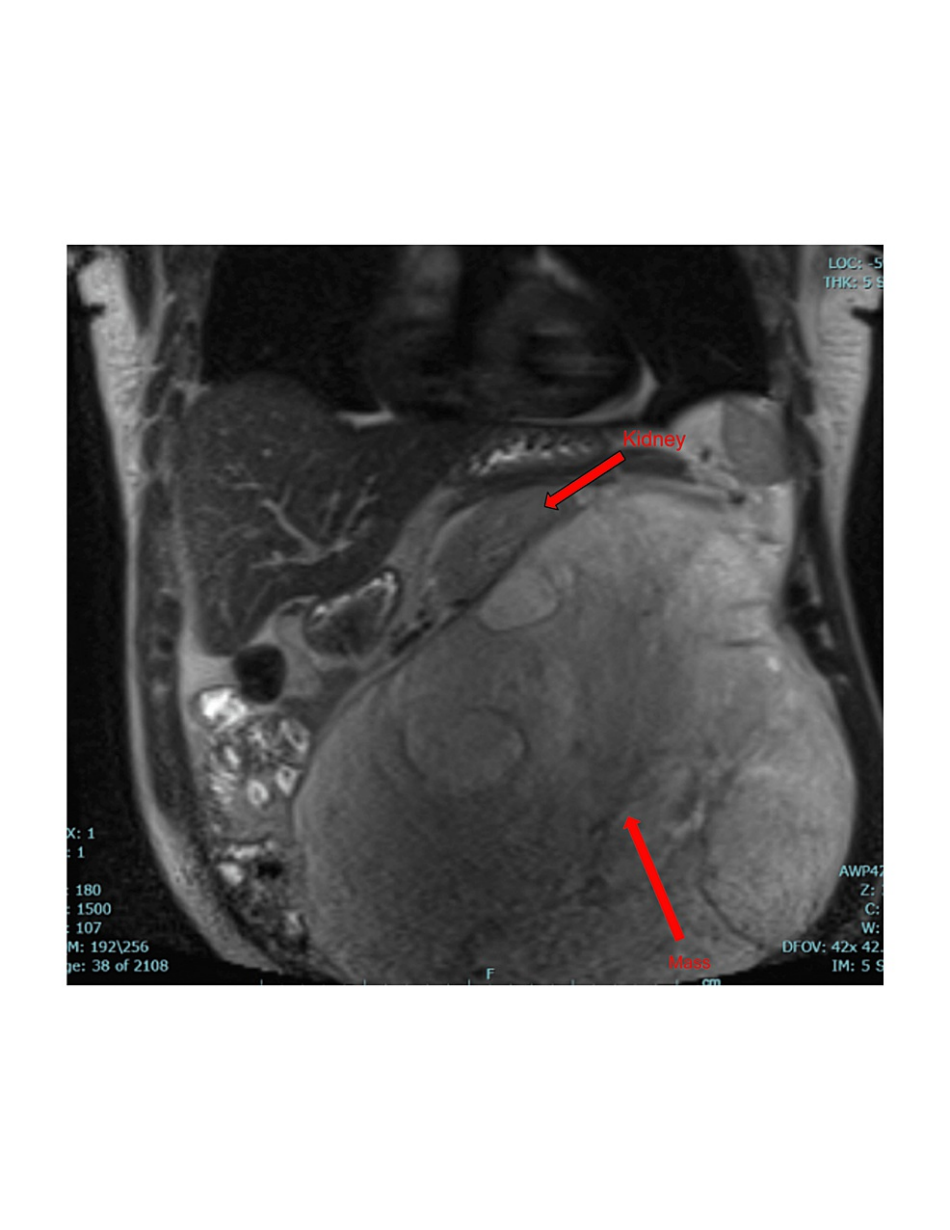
MRI of abdomen status post embolization of left retroperitoneal fat-containing mass displacing the left kidney MRI: Magnetic Resonance Imaging
